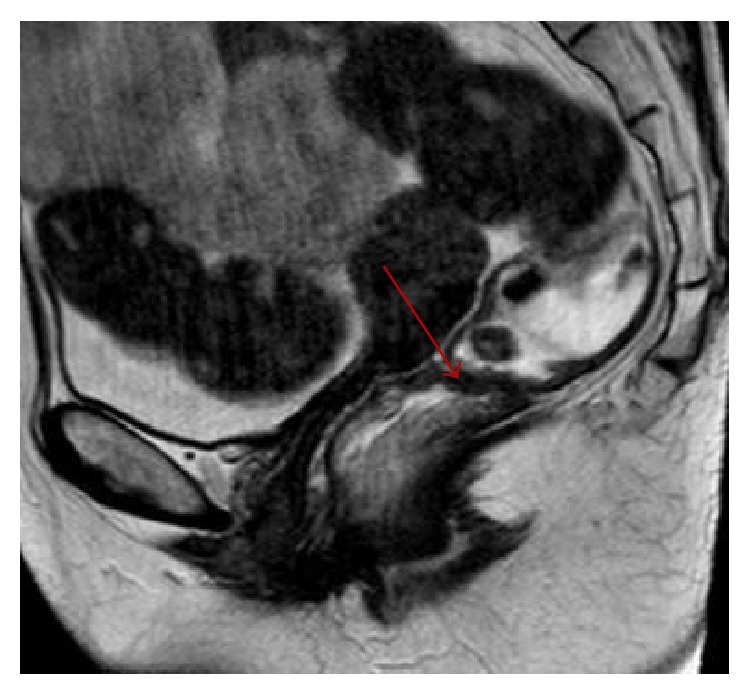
Postevacuating MR image showing thickening at the anastomotic line (arrow) and trapping of contrast in the segment cranial to it.
